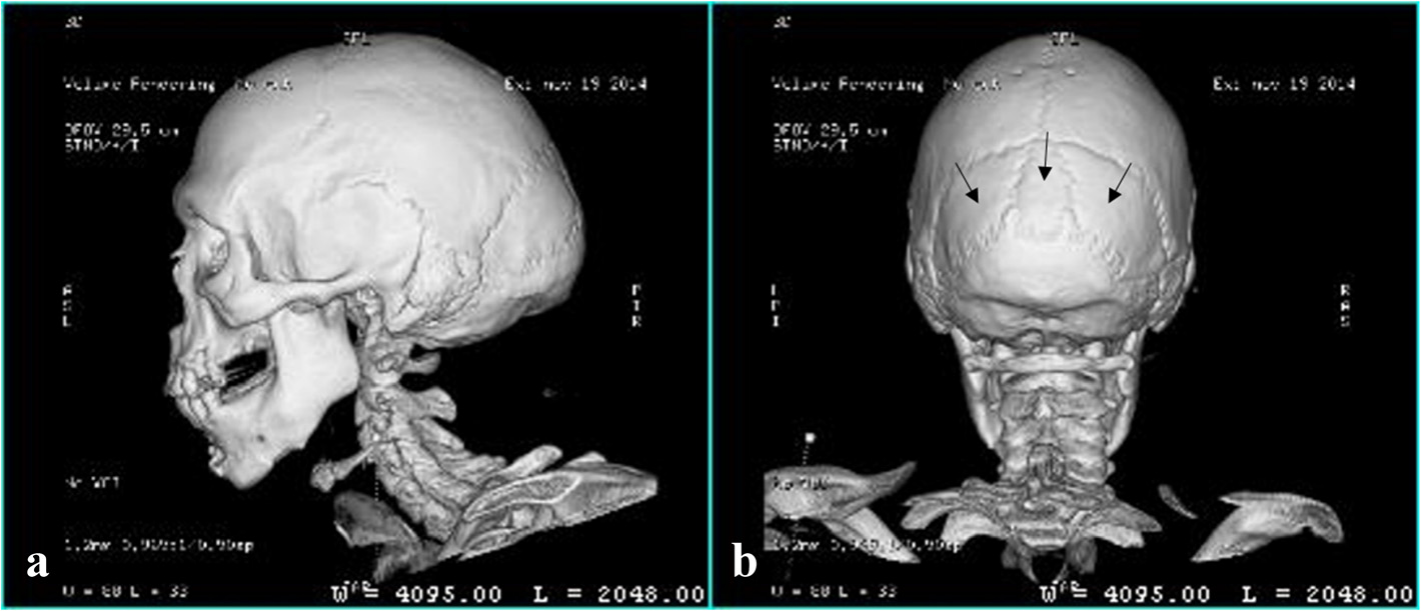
Three-dimensional reconstruction of the computed tomography of the patient’s head. a Lateral view shows surgical evidence in inferior maxillary bone, showing signs of microgenia, retrognathia, and a slight abnormality of dental occlusion. b Posterior view shows wormian bones in his occipital region
